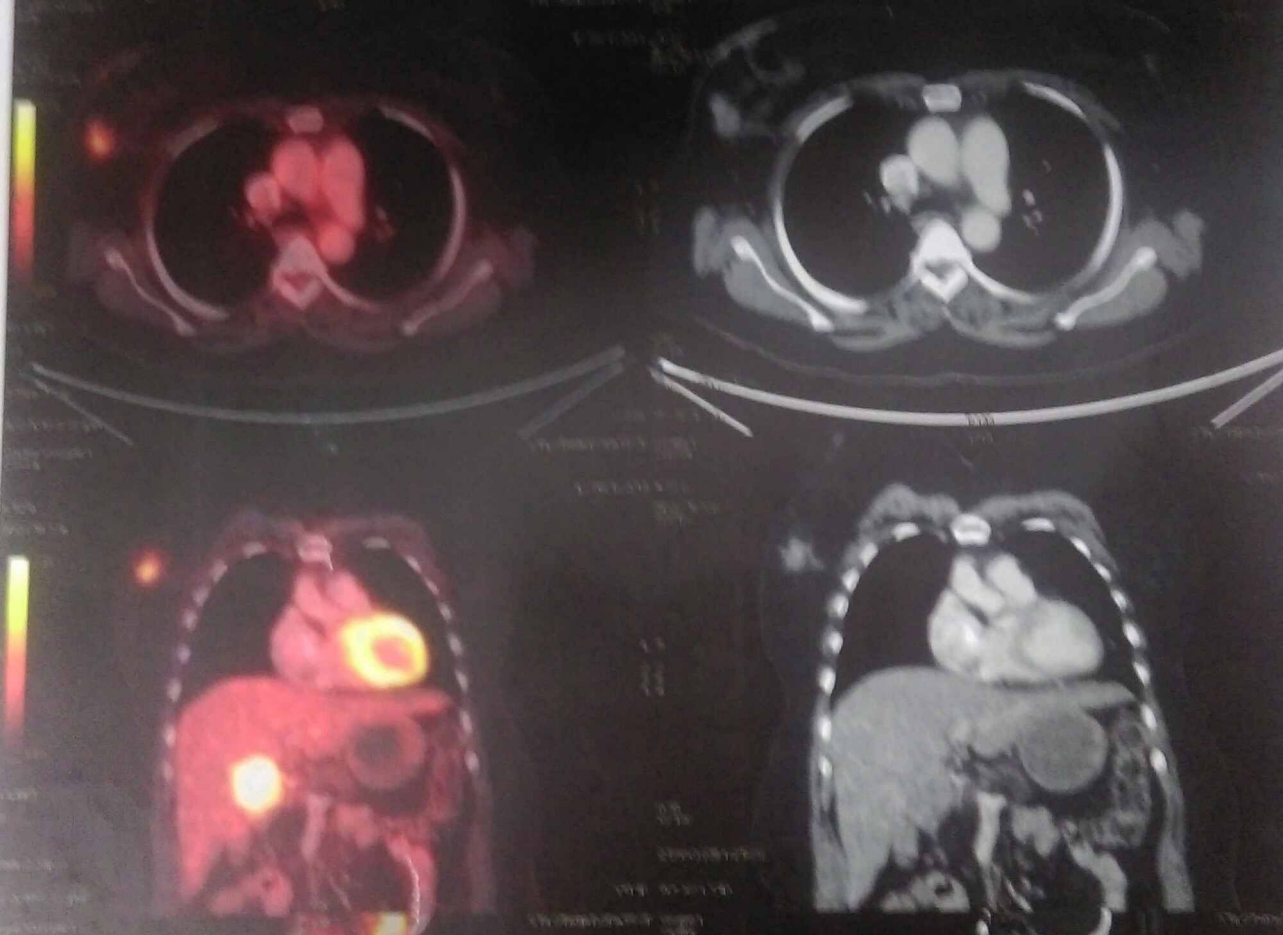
Whole-body PET-CT scan showing increased FDG uptake in the UOQ of the right breast of size 1.9 x 2.7 cm, SUVmax = 3.7 PET-CT: positron emission tomography-computed tomography; FDG: fluorodeoxyglucose; UOQ: upper outer quadrant; SUVmax: maximum standard unit value
